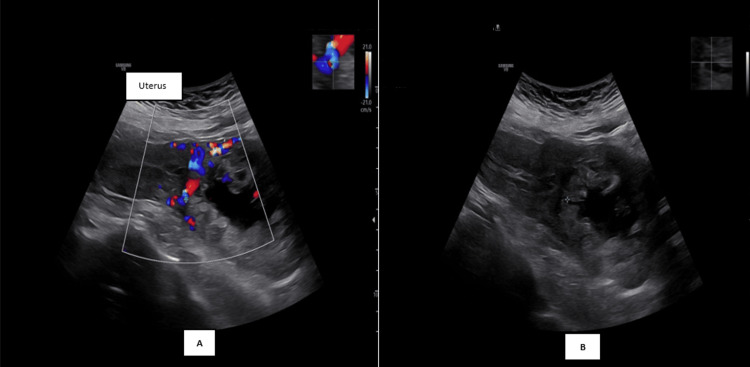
(A) Transabdominal ultrasound Doppler image showing an abnormal, highly vascularized sac located on the lower pole of the uterus. (B) Transabdominal ultrasound image without Doppler
